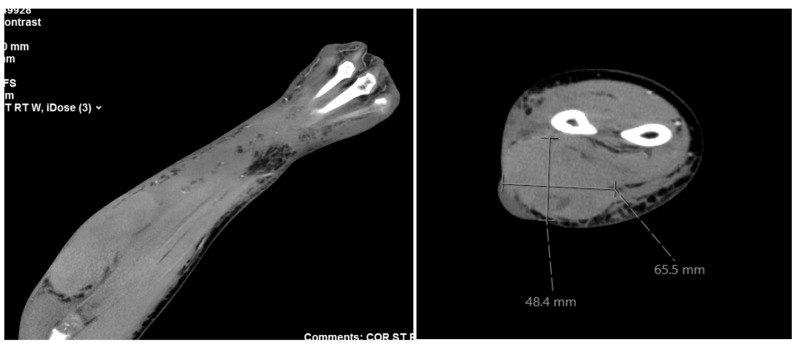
Pre-operative CT demonstrating a 4.8 x 6.6 x 13 cm fluid collection between fascial layers of the right forearm
